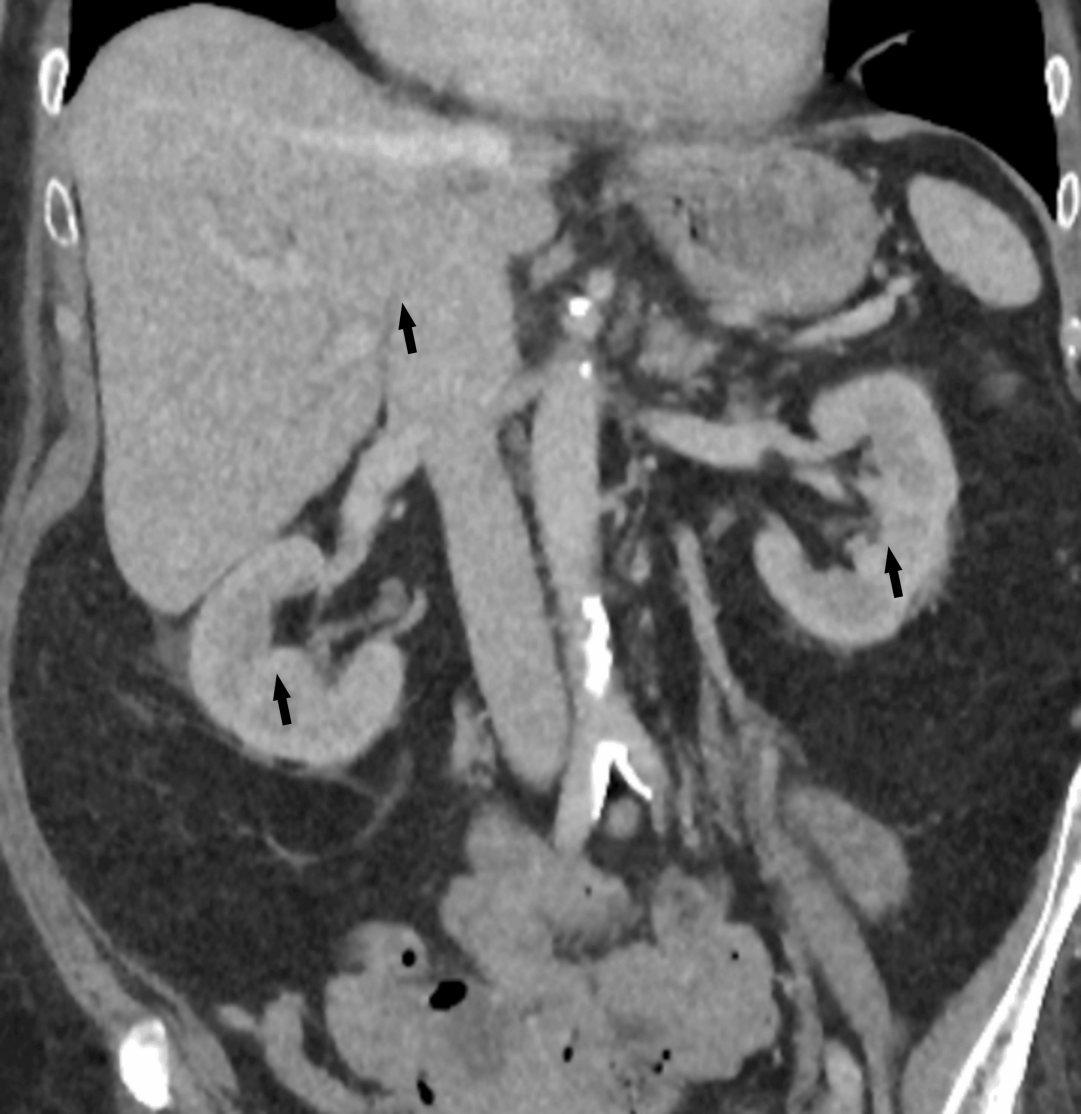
CT abdomen and pelvis with contrast CT Abdomen and pelvis with contrast showed no deep collection, no significant peri-nephric stranding, and no biliary infection. See black arrows.
